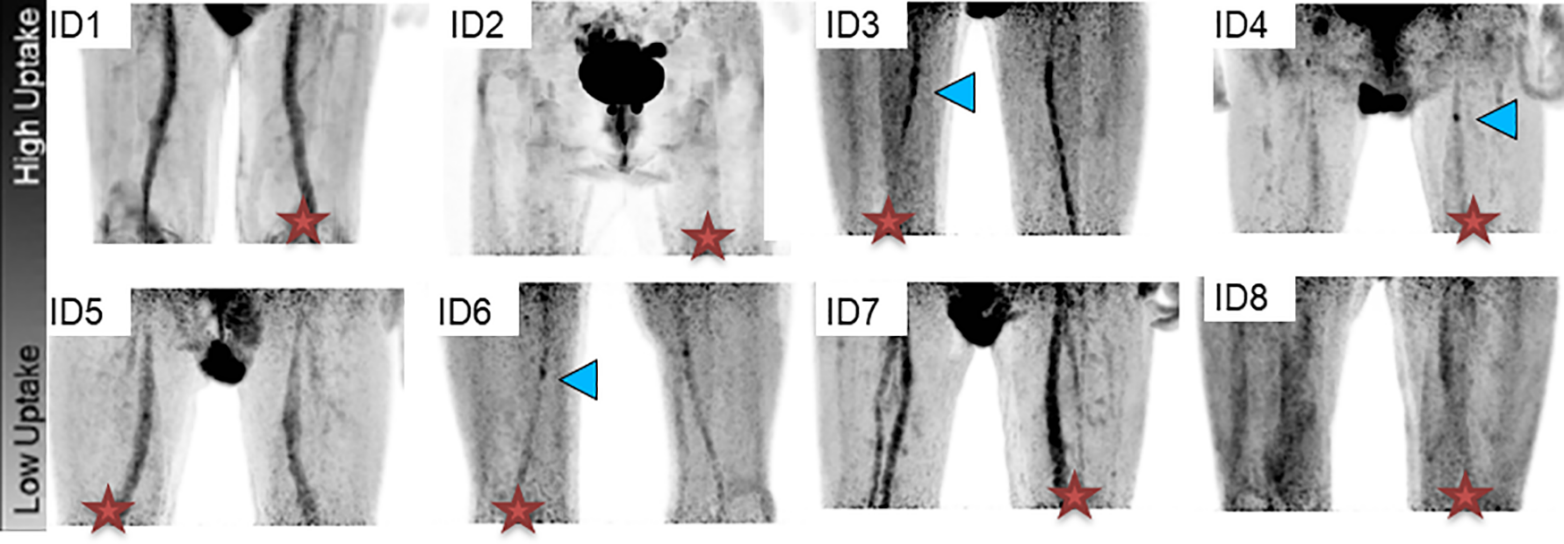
PET imaging results. Coronal views of 18F-FDG PET maximum intensity projections of the entire study population demonstrated different FDG uptake patterns among patients. A cross indicates the symptomatic side. Quantitative analysis [target-to-background ratio normalized to the muscle ROI signal (TBRm)] showed uptake (TBRm > 1) for all patients, except for patient ID 2.
